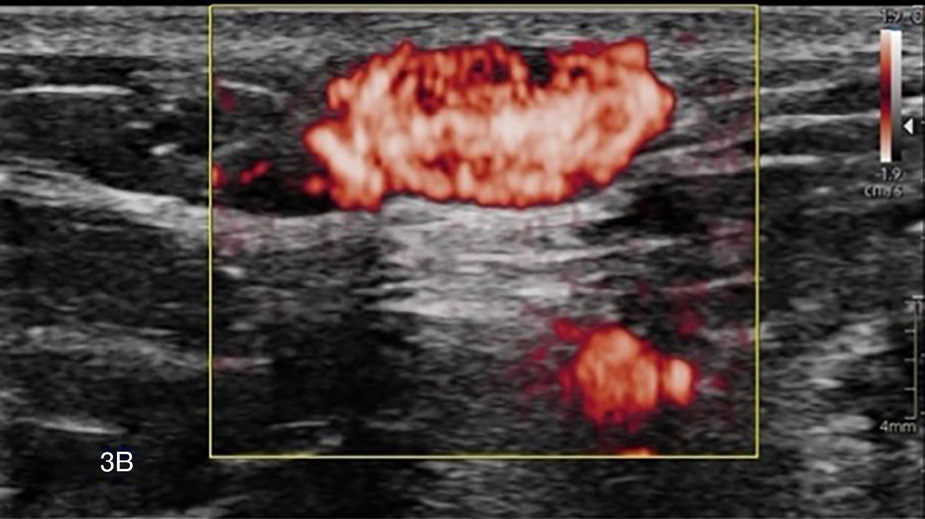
There is retro‑acoustic enhancement and high vascularization on color Doppler.
